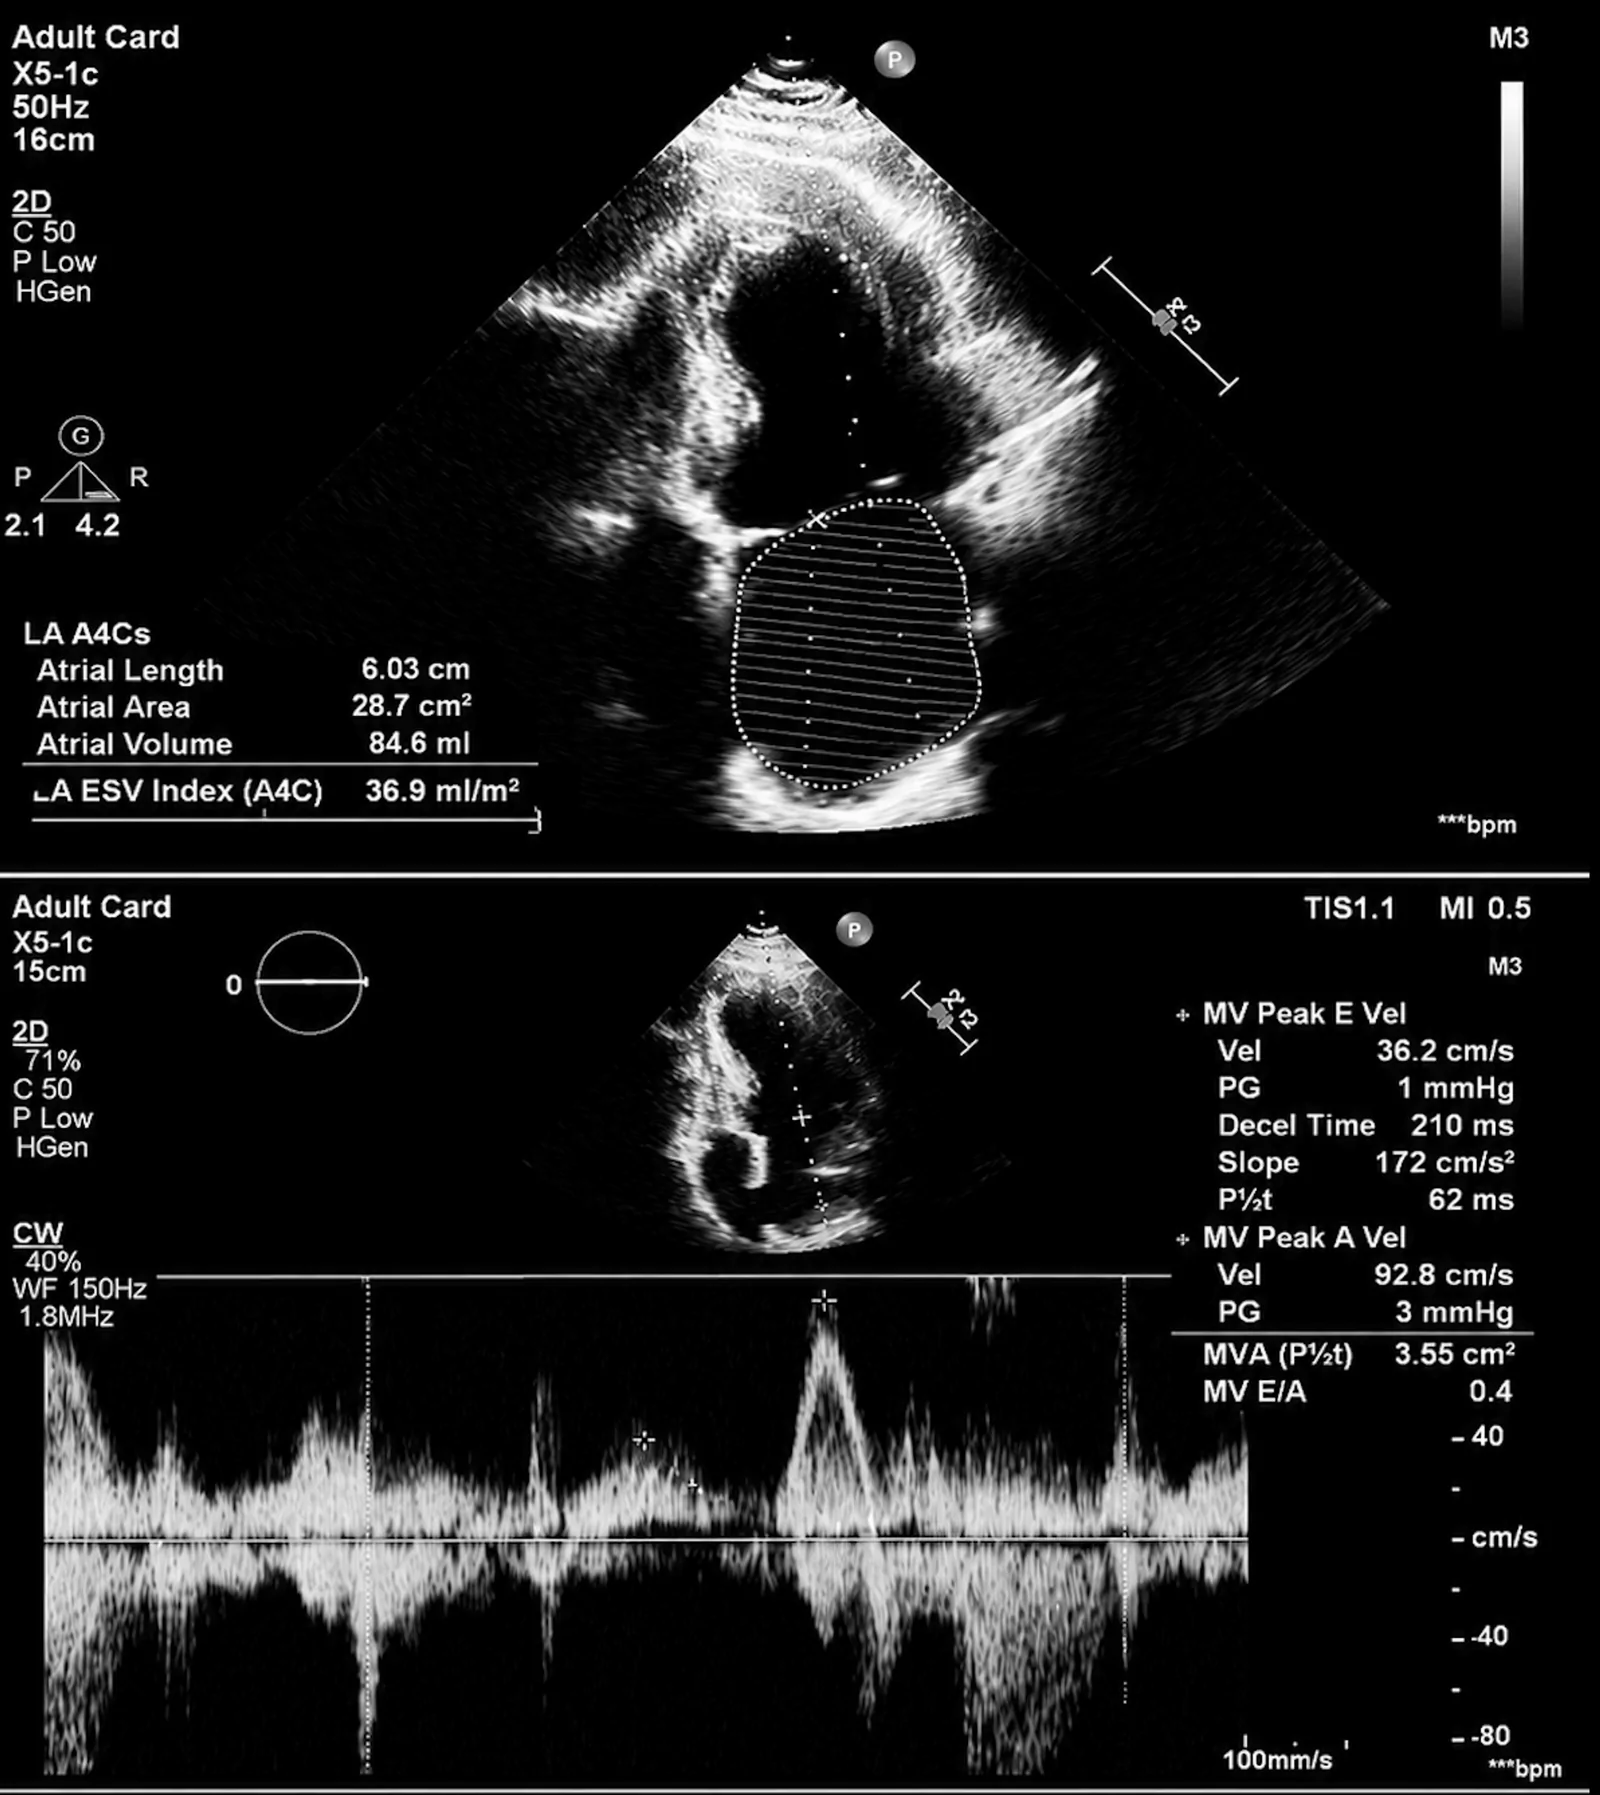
Transthoracic echocardiography showing left atrial volume index (LAVI) and mitral inflow velocities.
